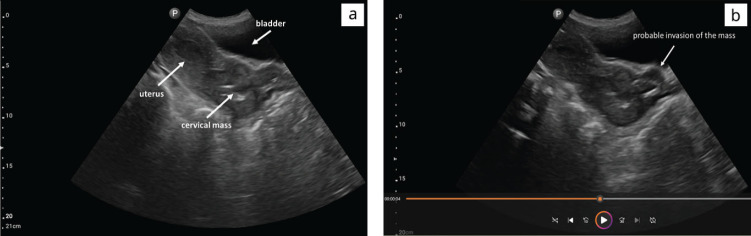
B-Modus sagittal plane of a female pelvis. (a) Ultrasound images show the cervical mass with probable extension beyond cervical margins (b).
